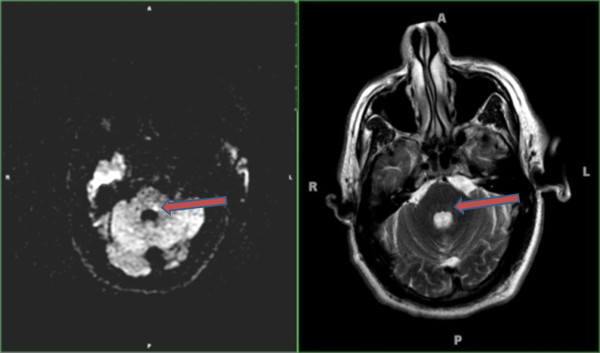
Axial diffusion-weighted MRI and T2-weighted MRI scans. On the day of presentation, axial diffusion-weighted MRI (left) showed a tiny focus of restricted diffusion in the left dorsal pons (arrow). Also, there was a corresponding subtle focus of signal hyperintensity noted on the T2-weighted fluid-attenuated inversion recovery sequence, which suggests a very tiny 4 mm infarct.
